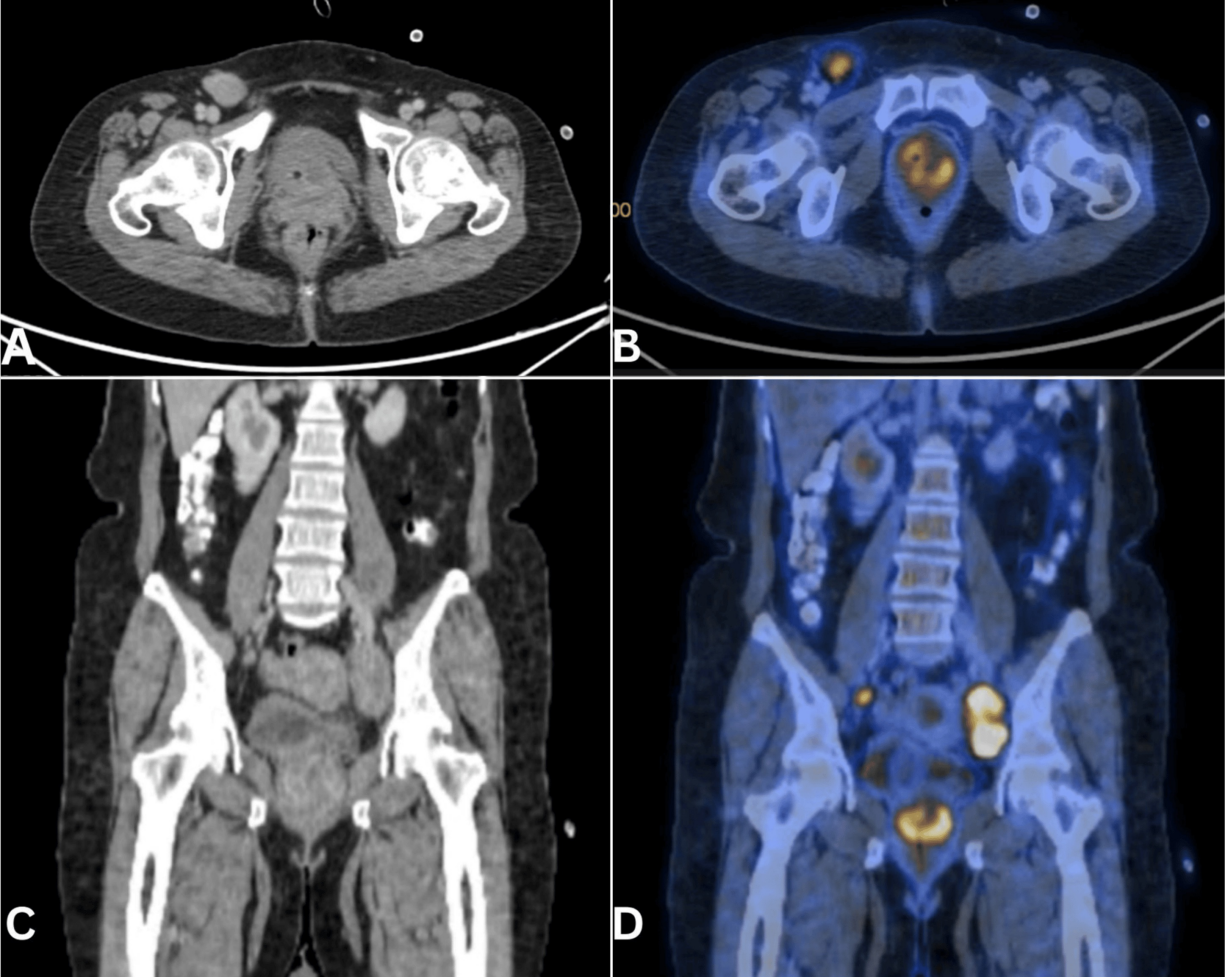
Axial (A) and coronal sections (C) of the CT pelvis with urethral tumor. Axial (B) and coronal (D) sections of PET CT showing metabolic activity CT: computed tomography, PET: positron emission tomography
